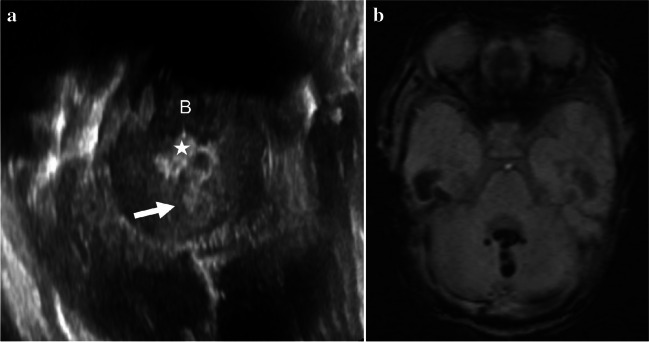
Representative case of type 4 cerebellar hemorrhage in a preterm boy (26 + 4 weeks, 530 g). a Axial left mastoid view of cranial ultrasound (rotated 90° clockwise to correspond with the axial MRI) shows cerebellar vermian hemorrhage (*arrow*) and echogenic intraventricular hemorrhage (*star*) in the fourth ventricle. b Axial susceptibility-weighted magnetic resonance image shows matching dark signal vermian hemorrhage and intraventricular hemorrhage. B brain stem
